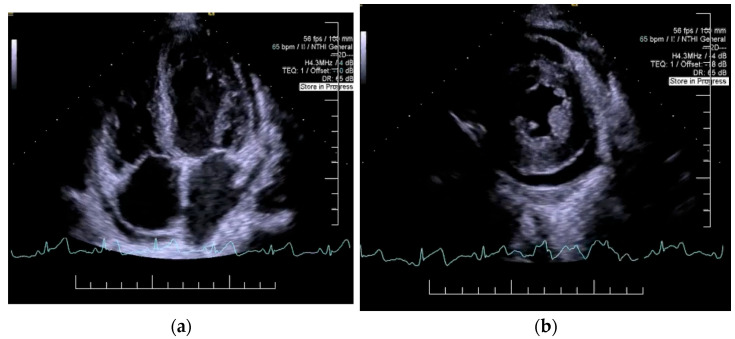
Echocardiography: left ventricular hypertrophy, impaired relaxation-type 1 diastolic dysfunction, (a) left ventricular ejection fraction of 50%, (b) circumferential pericardial effusion (9–11 mm).
